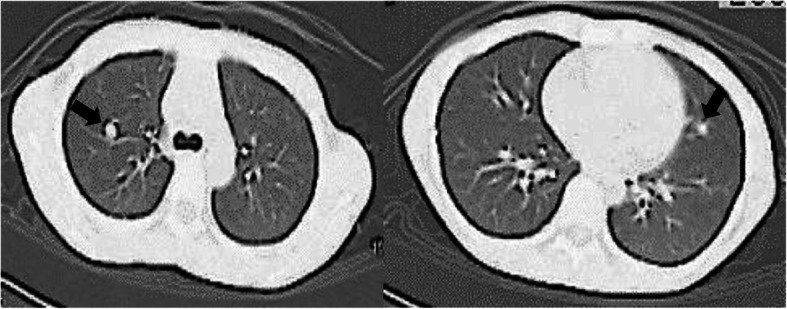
Chest plain computed tomography scan showed pulmonary nodular shadows of the S1 region in the right lung and lingular region in the left lung
